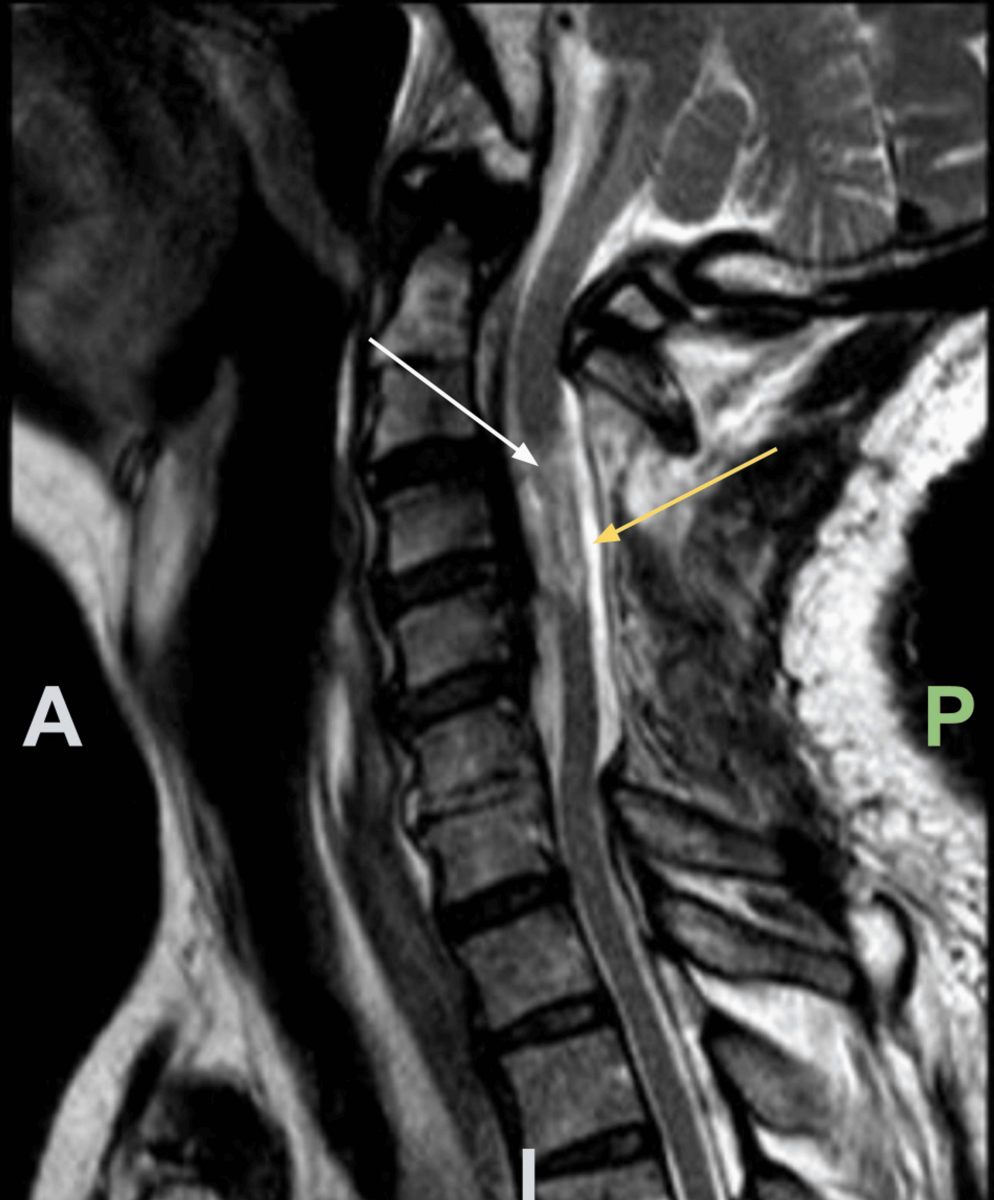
Sagittal view of postoperative cervical MRI, T2-weighted. An abnormal intramedullary T2 hyperintensity spanning from C3-C4 is seen (white arrow). CSF signal surrounding the spinal cord suggests adequate decompression (yellow arrow). A: Anterior, P: Posterior, I: Inferior
